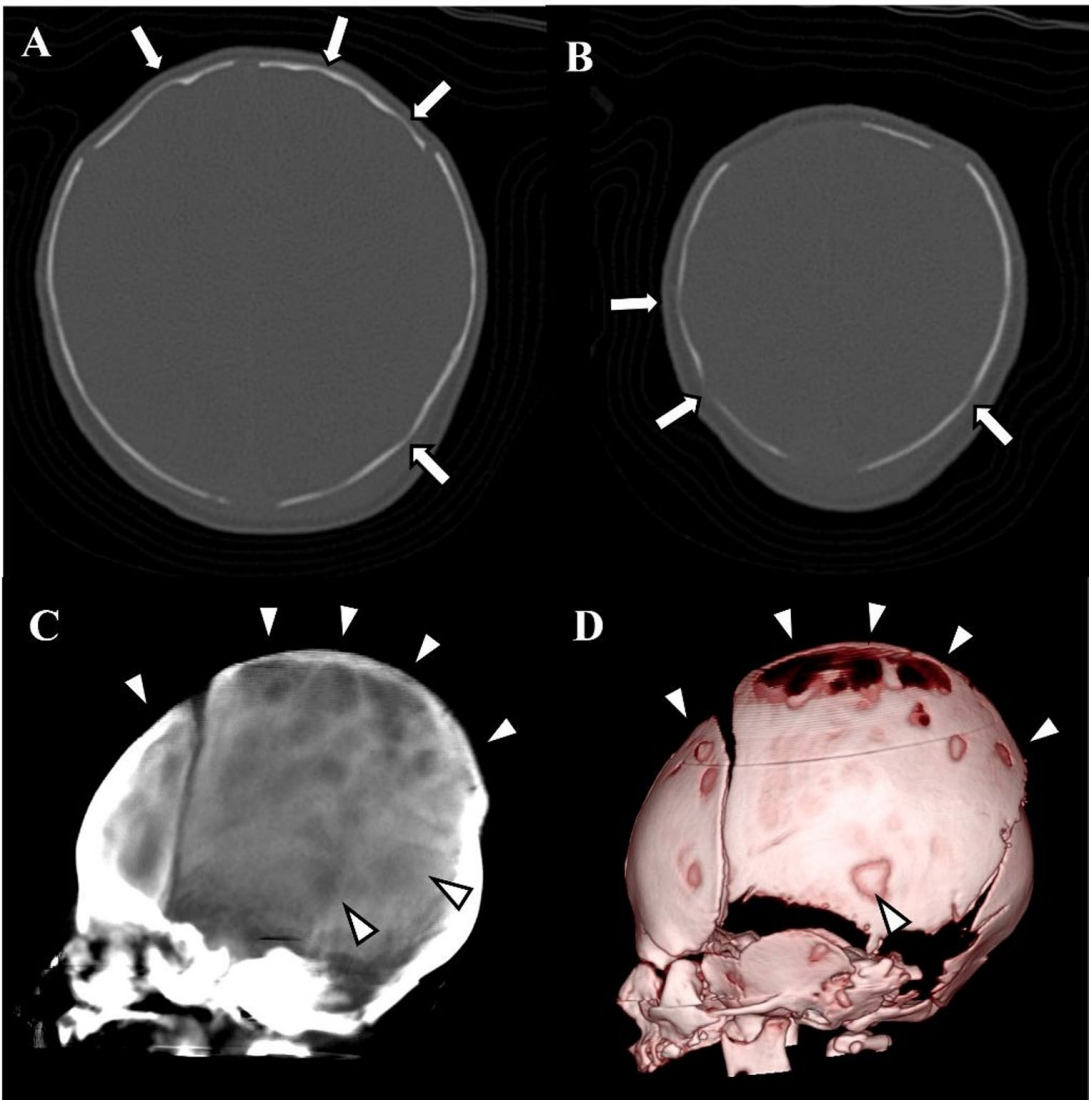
A zero-day-old boy with head trauma, showing no traumatic changes on CT or ICH symptoms
